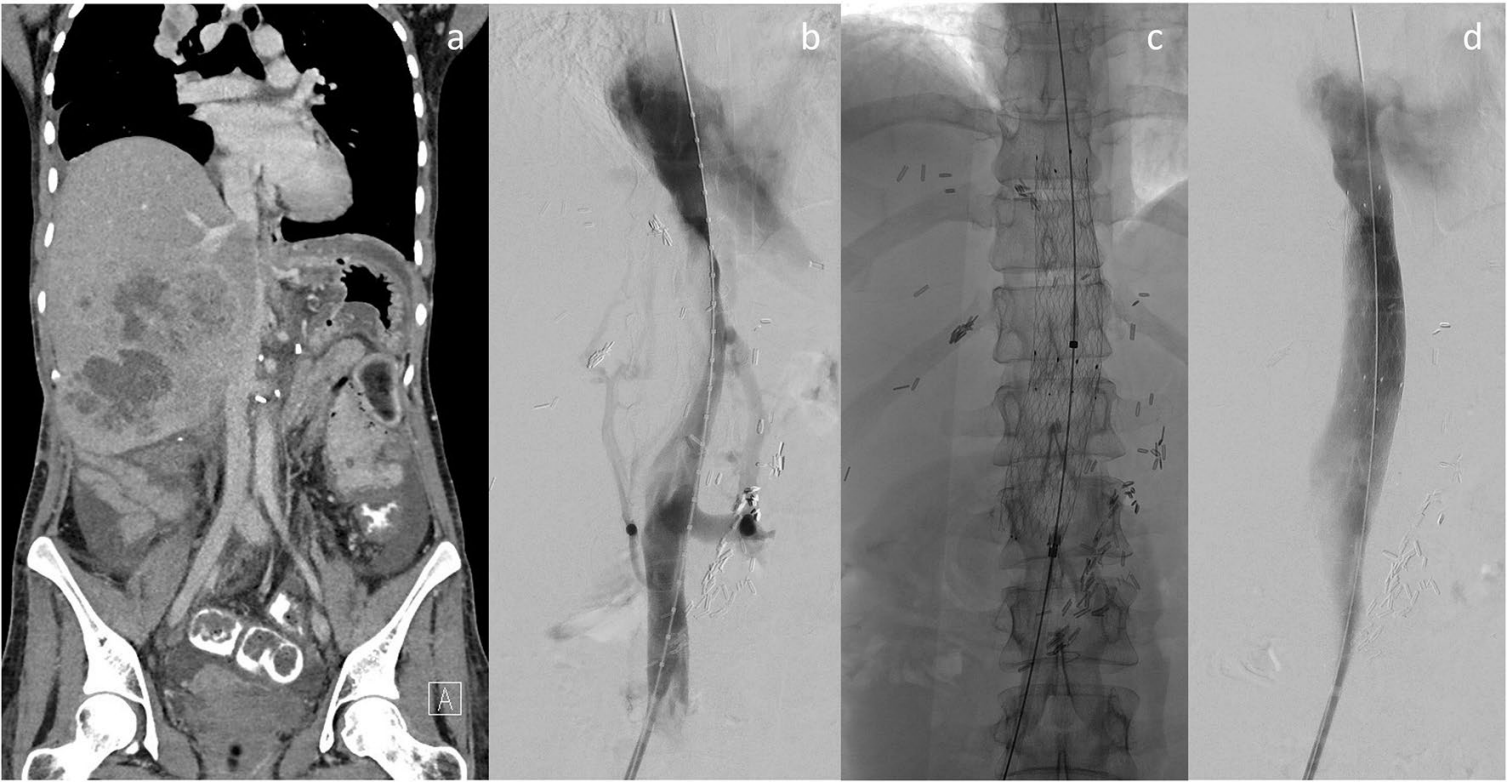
25-year-old female patient with hepatic metastasis of a retroperitoneal leiomyosarcoma. a Preinterventionally performed coronal contrast-enhanced computed tomography demonstrates high-grade stenosis of the IVC in the intrahepatic segment due to the intrahepatic tumor masses. b Corresponding cavography depicts the extent of the central venous obstruction and collateralization. c Fluoroscopic approval of the successful deployment of the Sinus-XL stent within in the malignant stenosis. d Cavography of the IVC after stent deployment demonstrates restored patency with reduced blood flow via collateral vessels
